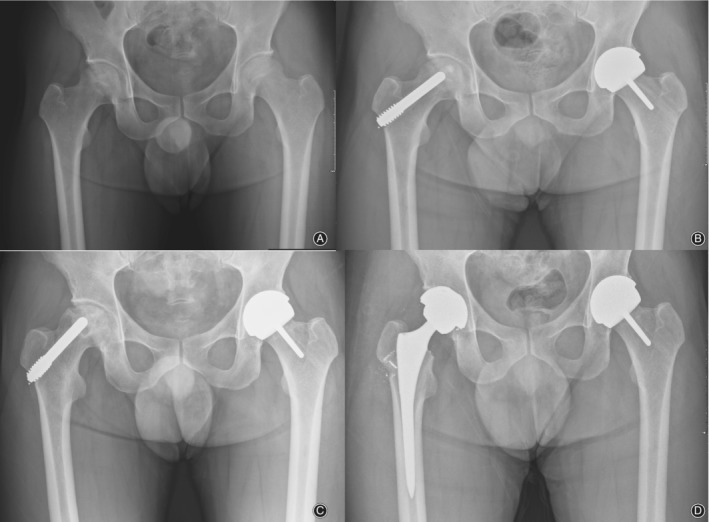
(A) Radiographs of a 46‐year‐old male patient with bilateral avascular necrosis of femoral head. (B) Postoperative radiograph showing that the right femoral head was underwent PTI and the left femoral head was underwent surface replacement. (C) Radiographs taken 12 months post operation show a progressive collapse of the femoral head of the right side. (D) The postoperative radiograph showed remaining metallic particles and bone loss at the lateral femoral cortex of the right side femoral head.
